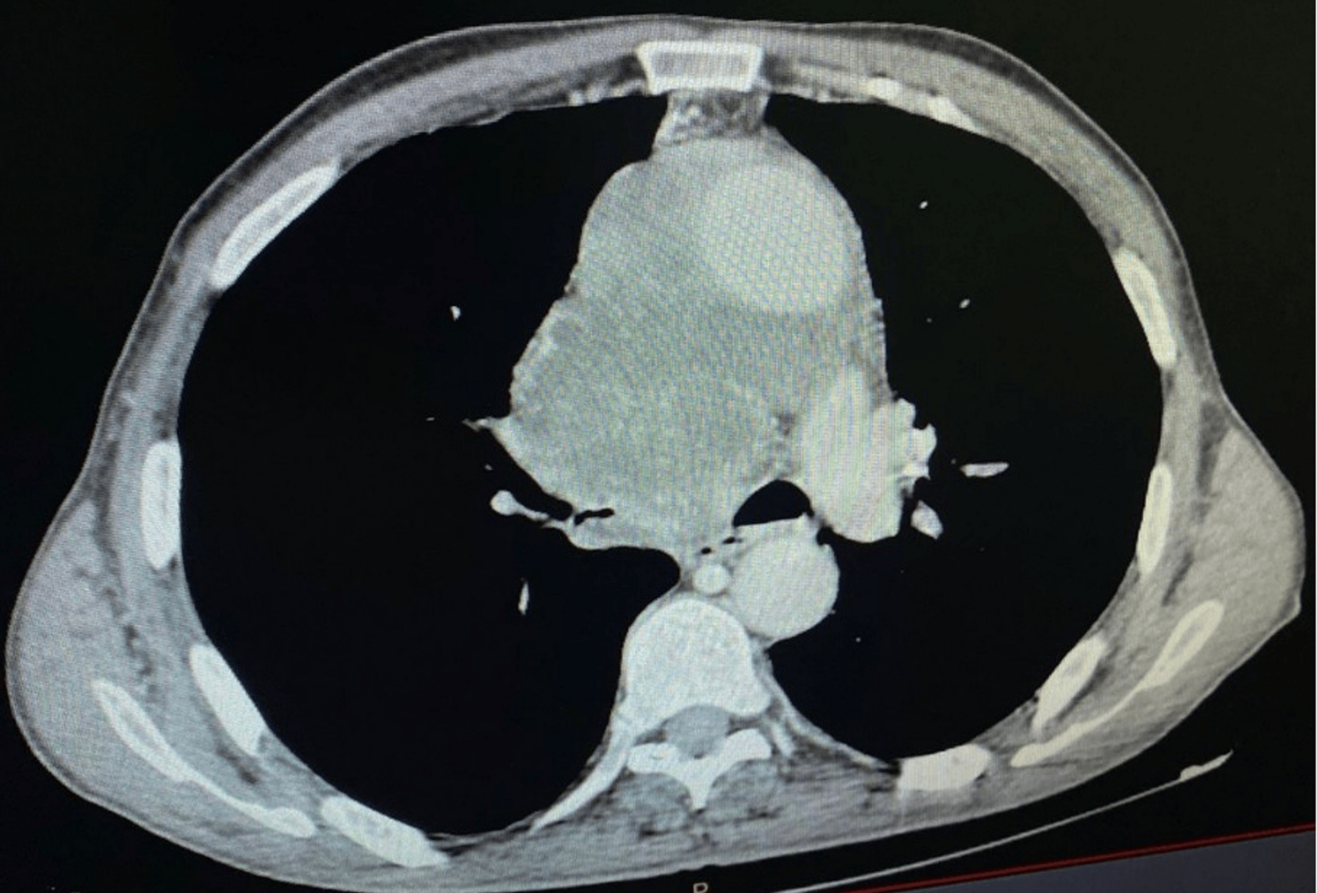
Chest CT scan showing an extensive mediastinal tumor mass with central necrosis and invasion of adjacent structures.
